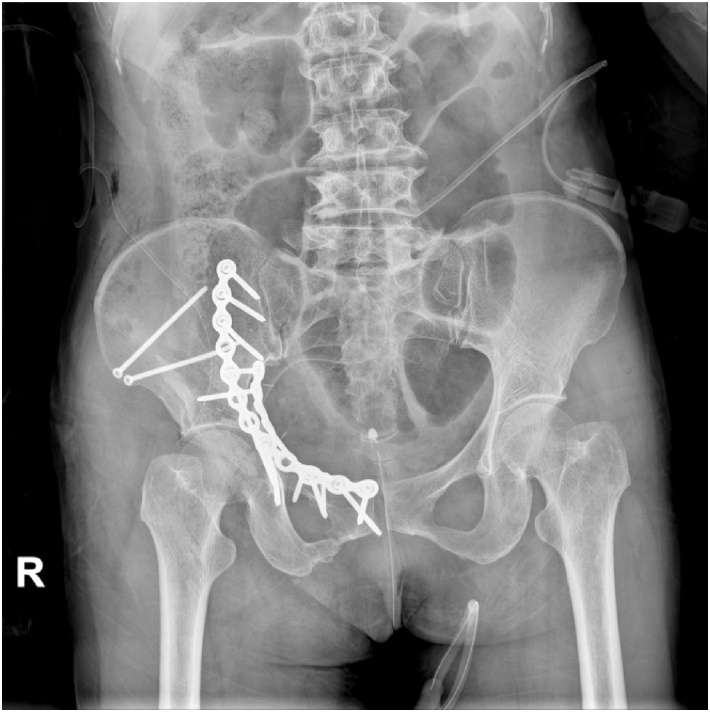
Post-operative radiograph of the pelvis showing near anatomical restoration of the fracture fragments with plates and cancellous screws.
